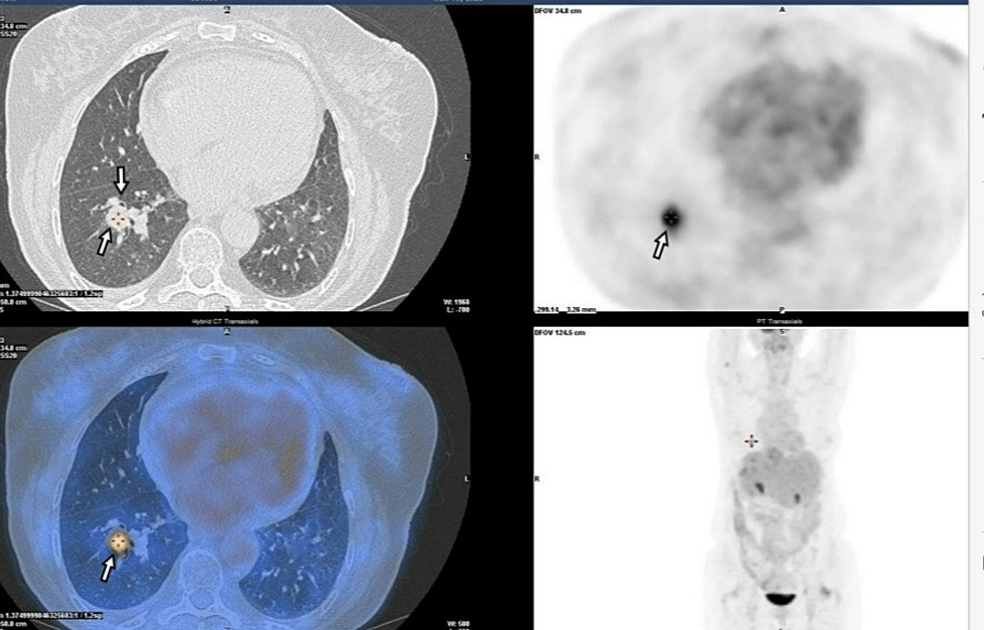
FDG-PET with mild uptake on the pulmonary nodule. The pulmonary biopsy was compatible with pulmonary anthracosis FDG-PET: fluorodeoxyglucose positron emission tomography
